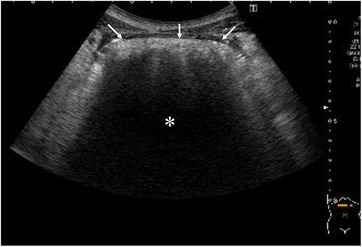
Ultrasonography findings. Ultrasonography showed a large intra‐gastric lesion with smooth borders (arrows) and a broad acoustic shadow (asterisk), causing an impossible evaluation of its inner structure.
